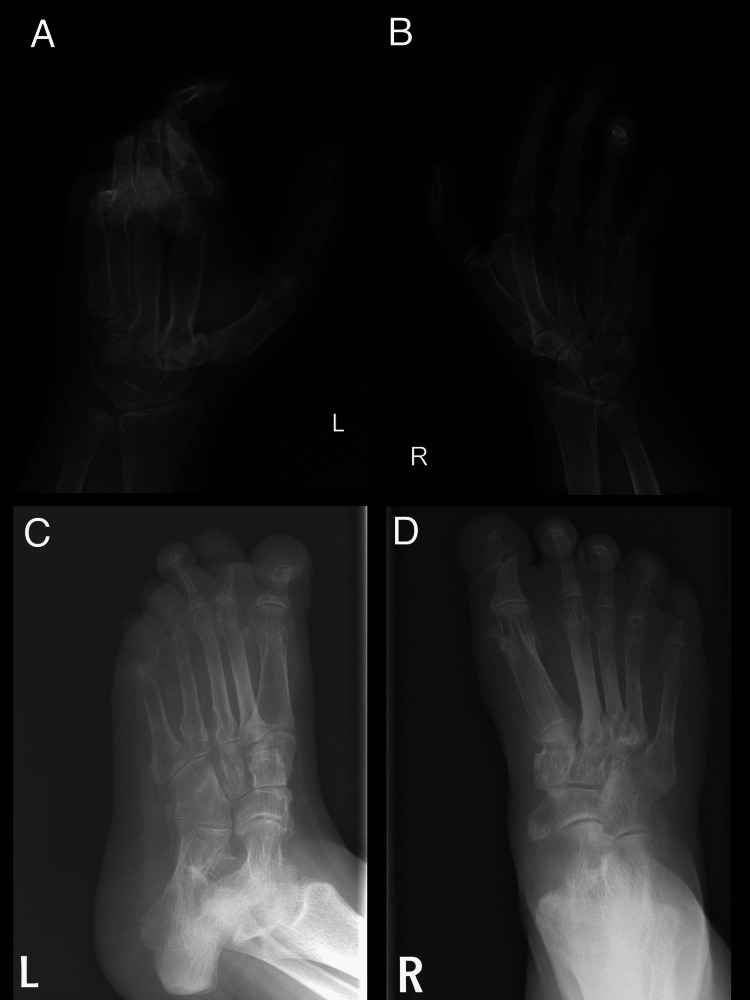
Radiographs of both hands and feet Radiograph of hands: A and B show marked osteopenia, flexion deformity of the interphalangeal joints more at the left hand, bony resorption of the distal part of fifth metacarpal bones and proximal part of middle phalanx of little fingers, Radiograph of feet: C and D show bilateral flat feet with marked osteopenia, destruction of interphalangeal joints of the big toes, fused proximal interphalangeal joints of the remaining toes, and increased bone resorption seen at distal tufts of proximal phalanges of left fourth and fifth toes.
